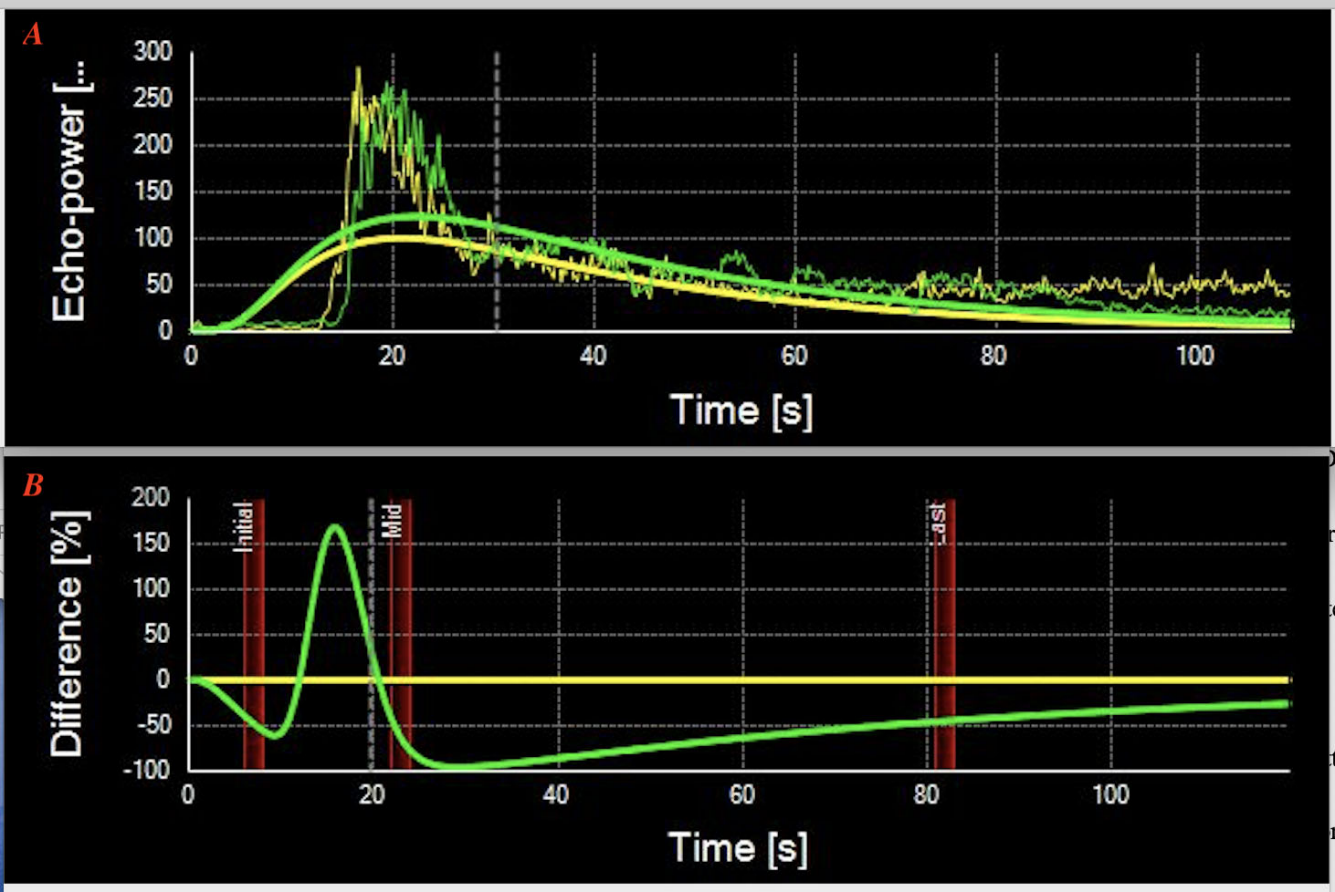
(A) Contrast-enhanced color Doppler ultrasonography-time–intensity curves (CDU-TICs) of the retinal pigment epithelium (RPE) neoplasms present a specific fast fill-in and slow washout-shaped curves (Patient 9). (B) Contrast-enhanced CDU-TICs of the uveal melanoma present with fast fill-in to fast washout-shaped curves.
